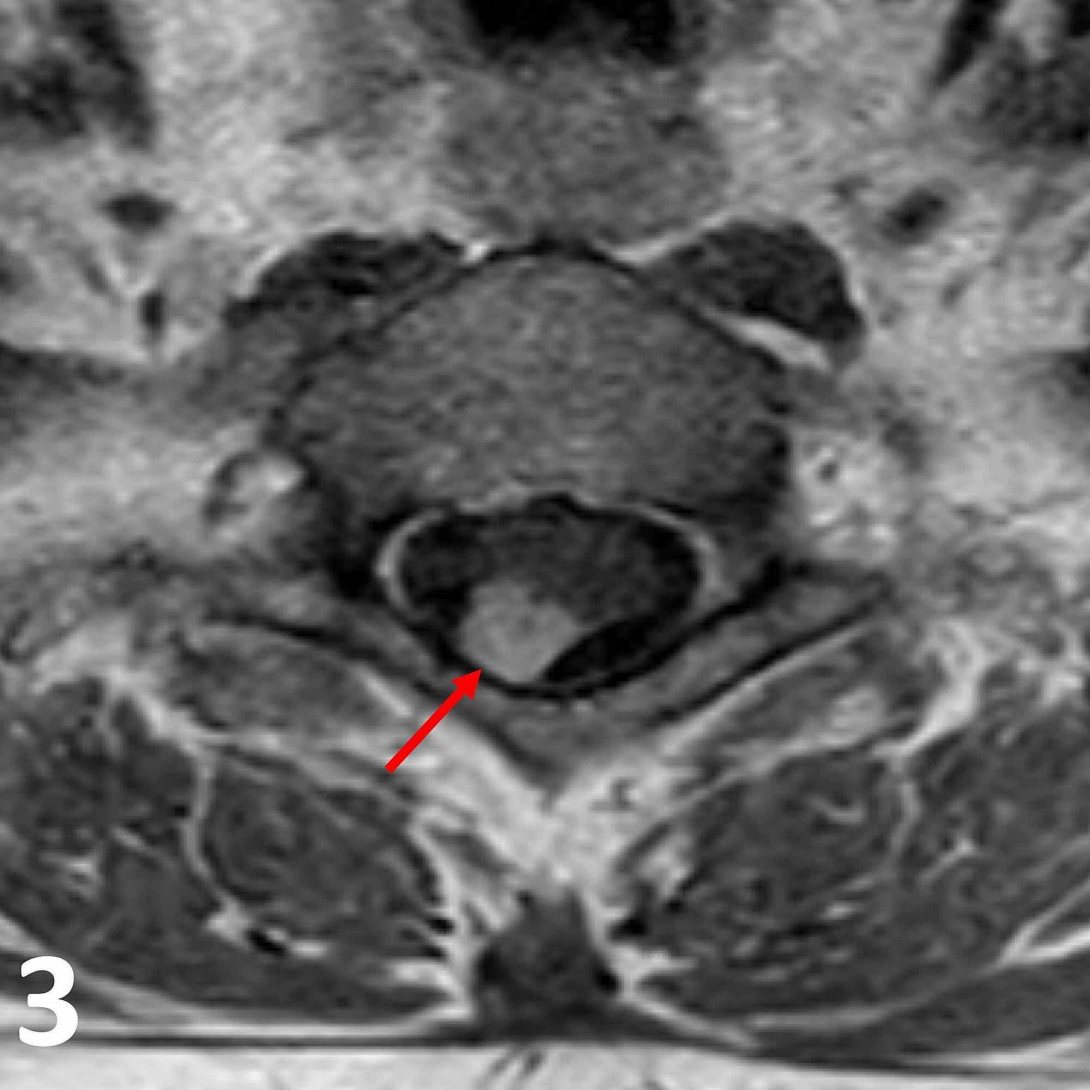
Axial T1 post-gadolinium MRI image at the T1 vertebral level. Note the homogenously enhancing intradural lesion (red arrow) in the right posterolateral aspect of the spinal cord. The lesion exhibits both intramedullary and extramedullary components, which are rarely reported in schwannomas.
